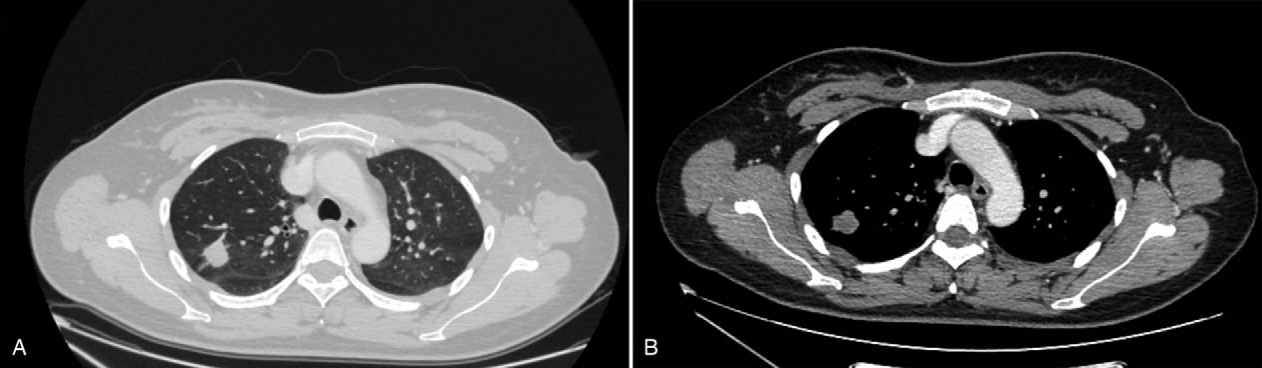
Computed tomography in patients with pulmonary inflammatory pseudotumor. Lung windows (A) and mediastinal windows (B) showing a right upper lobe tumor with speculation.
